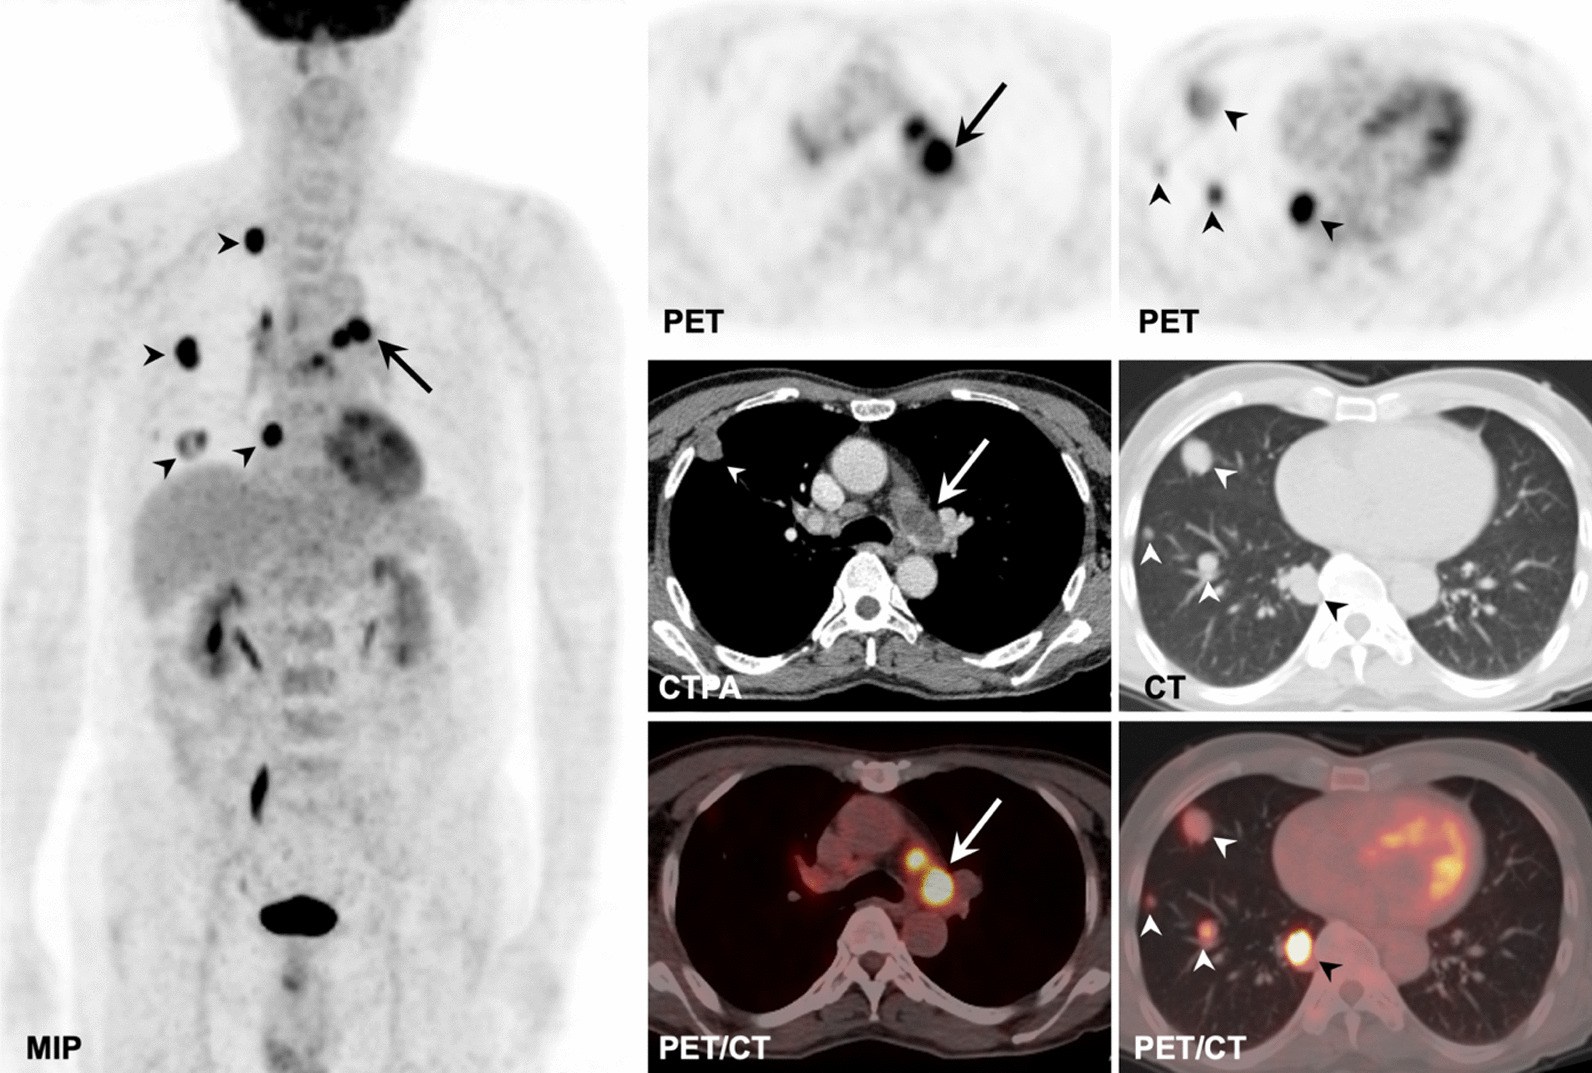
PAS in a 67-year-old man involving PT, RPA, and LPA (solid arrows) with SUVmax of 11.3 on the MIP, axial PET, and fused PET/CT images and filling defect on the CTPA image, with the metastases of right lung (arrowheads) on the MIP, axial PET, CT, and fused images and mediastinal and right hilar lymph nodes
